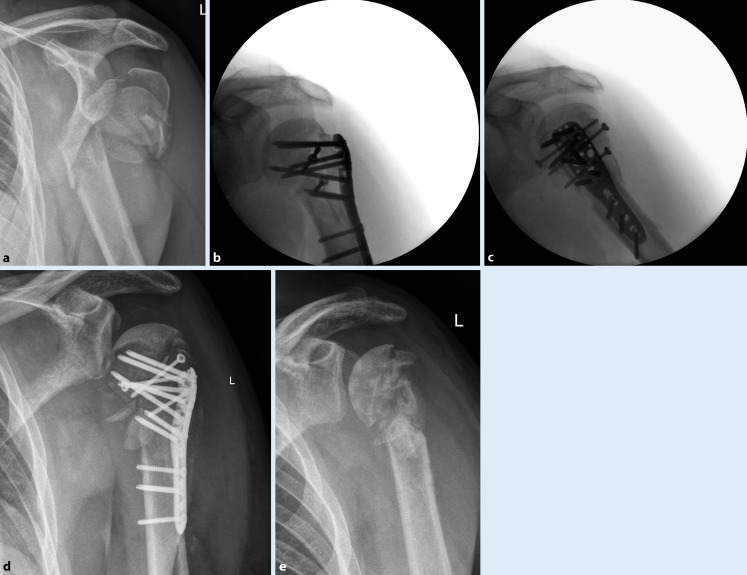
A 50-year-old patient with a dislocation fracture type 11C3 on the left side (a). Surgery was performed 3 days after trauma. Intraoperatively, the humeral head was completely dislocated from the glenoid. The intraoperative radiographs confirmed an anatomical fracture reduction (b, c). After 3 months, the patient complained of pain on movement and the radiograph showed a stage III avascular necrosis of the inferior part of the humeral head (d). The patient underwent revision surgery with implant removal alone (e)
